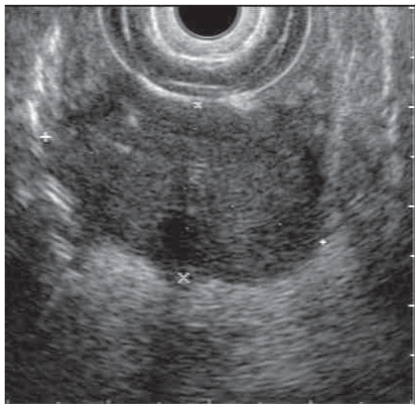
Endoscopic ultrasound of duodenal mass.
